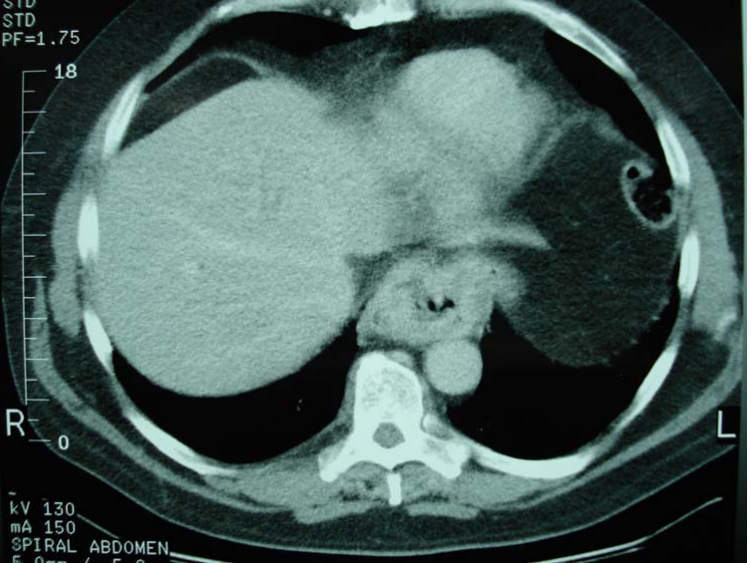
Initial CT scan revealed a large mass in the distal third of the esophagus.
